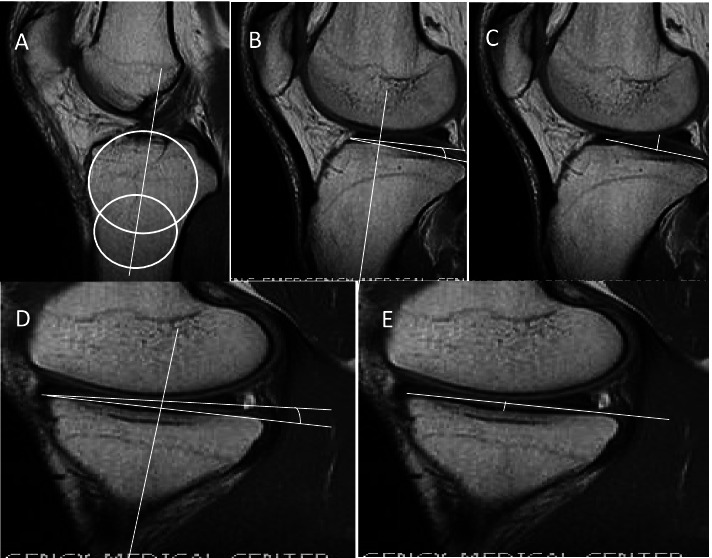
As described previously, various indicators of bone morphology were measured by MRI: A midsagittal tibial reference, B lateral tibial slope (LTS) [13], C lateral tibial height (LTH) [14], D medial tibial slope (MTS) [13], and E medial tibial depth (MTD) [3]
